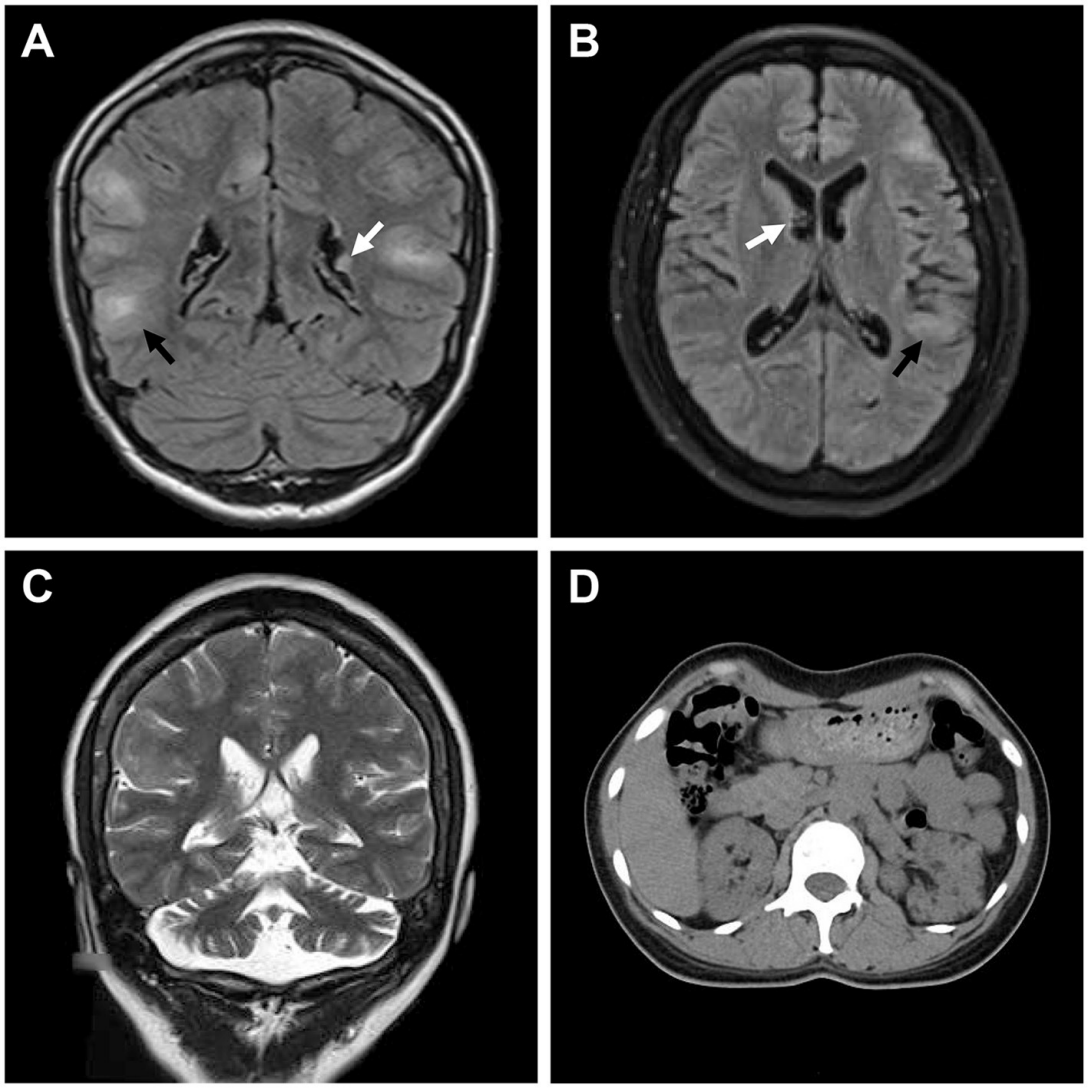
Medical images of patients with TSC. (A) Water-suppressed T2-weighted MRI sequence of the proband and (B) water and fat-suppressed T2-weighted MRI sequence of patient II:2, showing cortical dysplasia (black arrow) and subependymal nodules (white arrow). (C) T2-weighted MRI sequence of patient II:2, showing cerebellar atrophy. (D) CT scan of patient II:2, showing renal angiomyolipomas and cysts.
